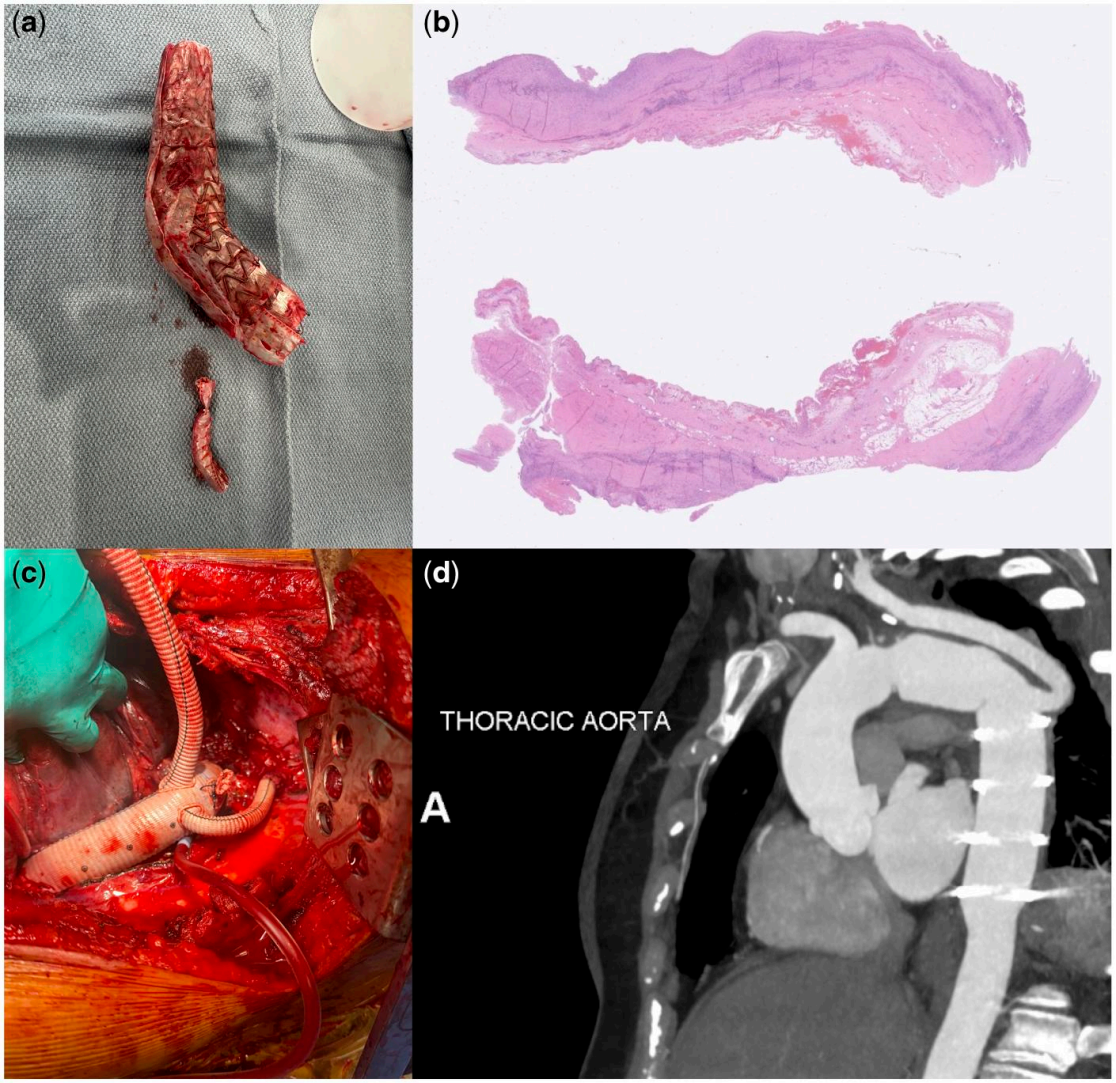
(a) Inflamed, explanted TBE; (b) pathologic assessment of resected aorta revealed marked acute and chronic inflammation and granulation tissue; (c, d) intraoperative photo and 2-month postoperative CTA demonstrating DTA and distal arch reconstruction. DTA: descending thoracic aortic; TBE: thoracic branched endograft
